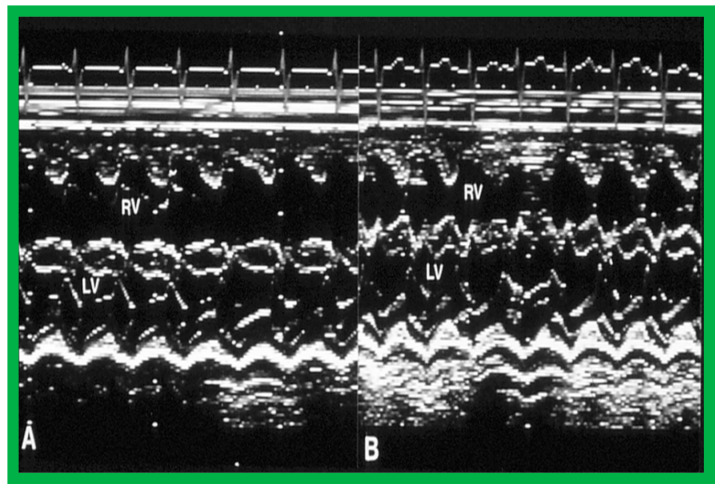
Selected m-mode tracings from parasternal long axis view prior to (A) and following (B) balloon pulmonary valvuloplasty, demonstrating a decrease in the size of the right ventricle (RV). LV, left ventricle. Reproduced from Reference [136].
